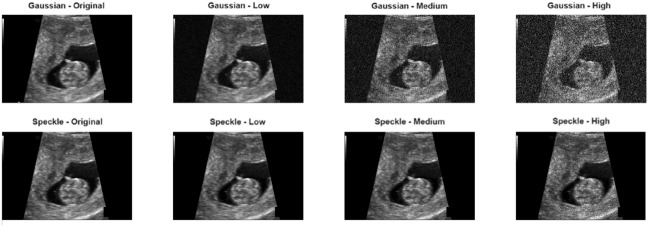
Noise types and with different intensities for fetal ultrasound dataset.
